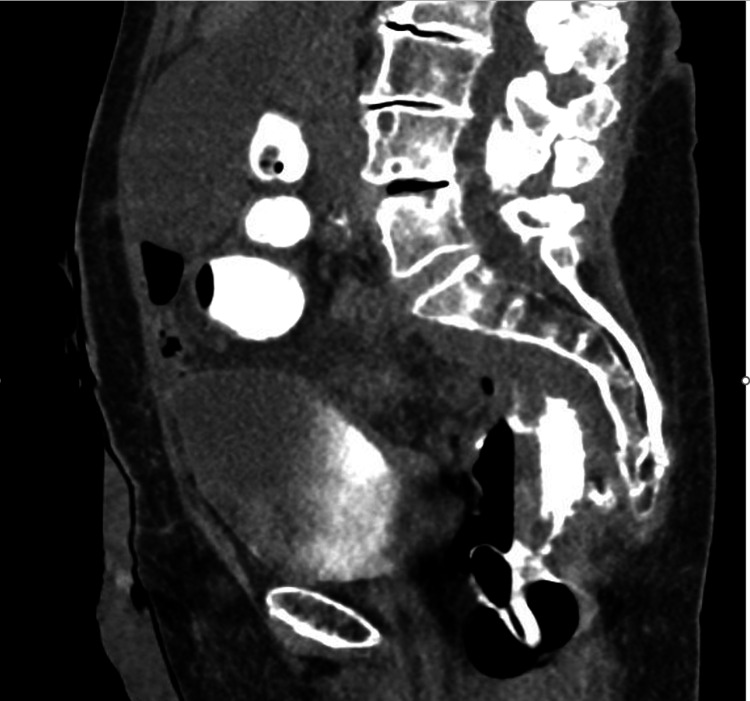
CT scan of the abdomen and pelvis with rectal contrast four months after surgery to evaluate for reversal. There is a persistent abscess cavity posteriorly seen with a small tract connected to the rectum. The tract and cavity were filled with contrast.
